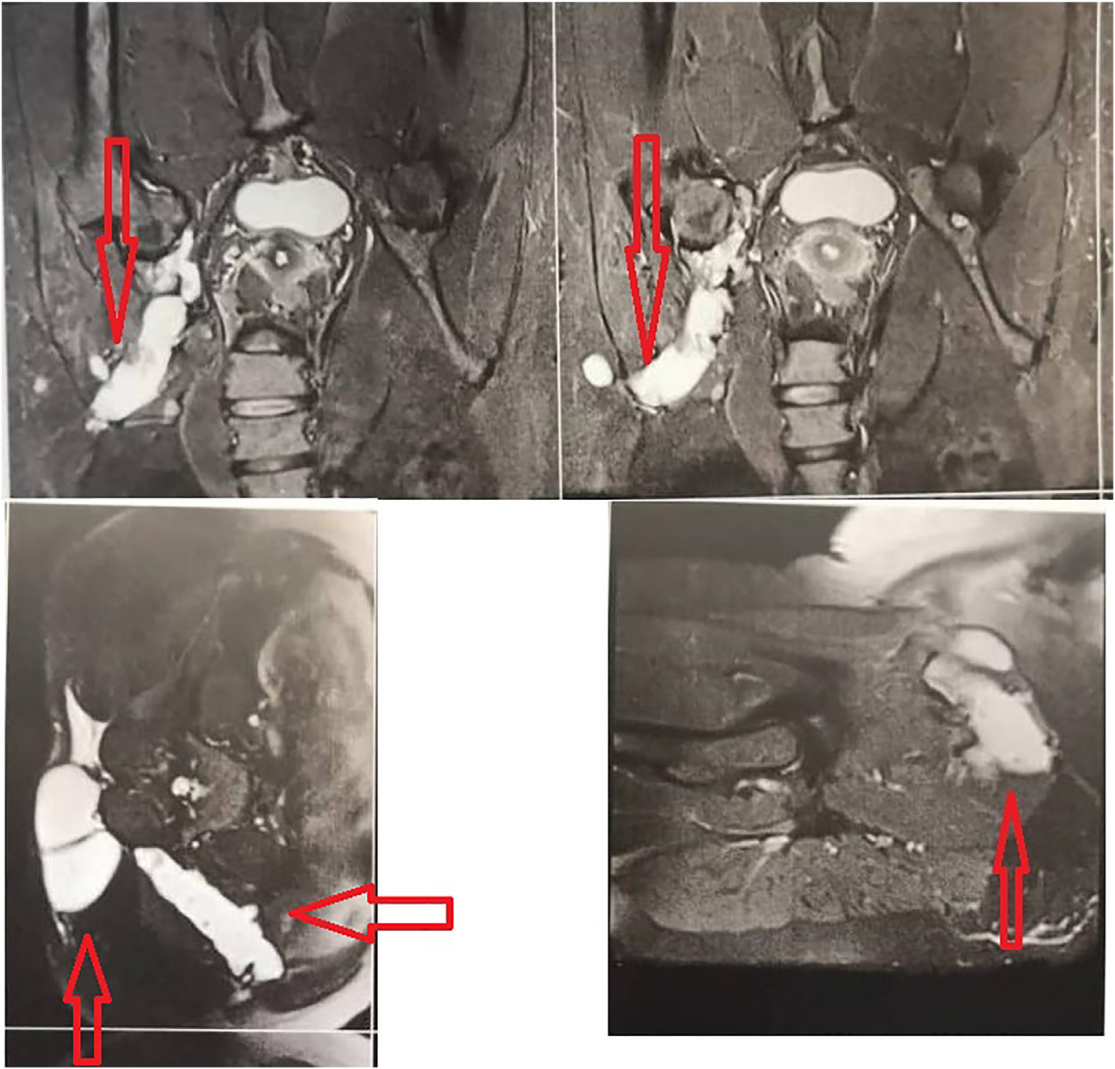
CT scan and MRI of the pelvis showing cysts (red arrows).
